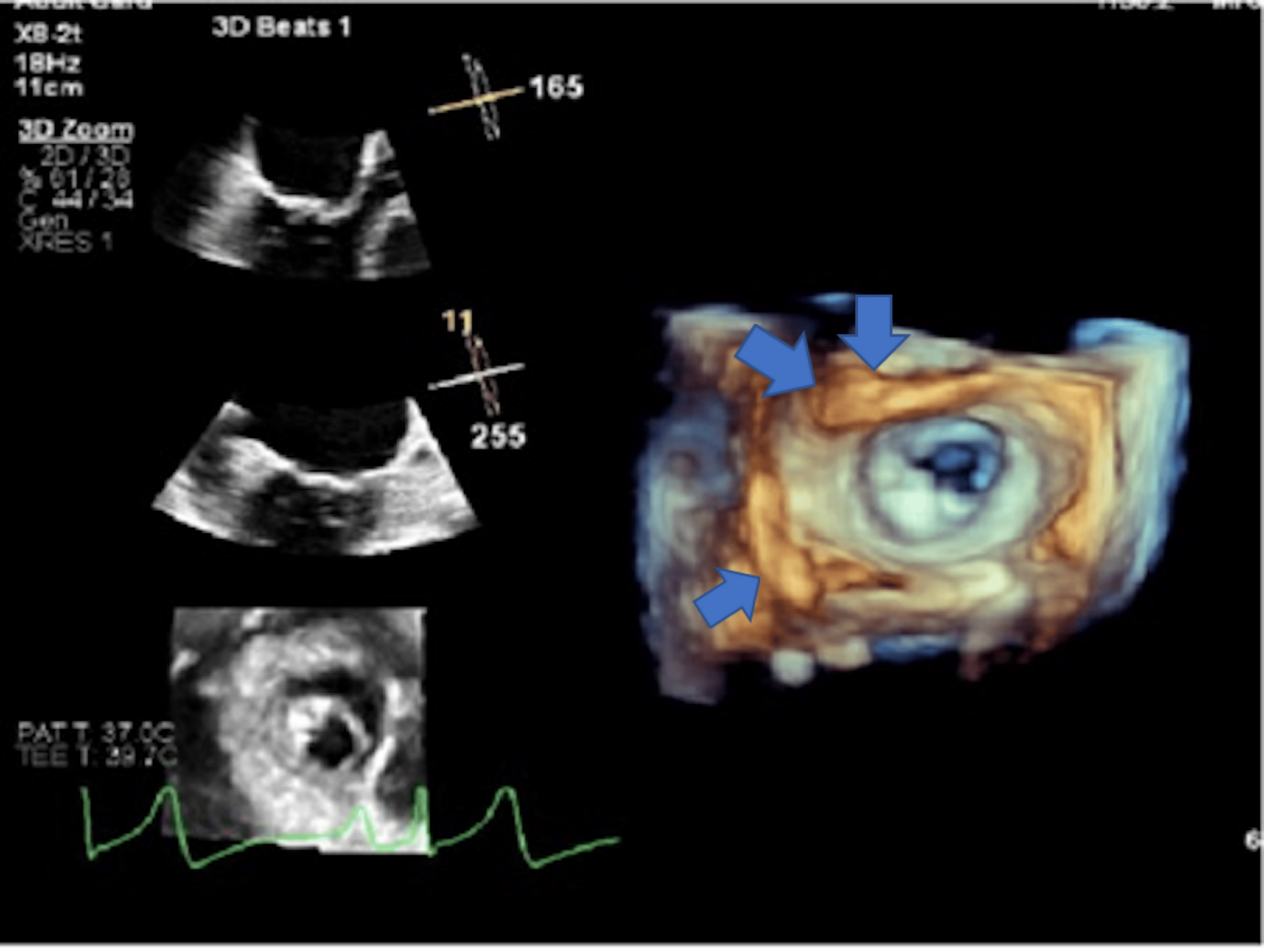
Transesophageal echocardiogram showing severe mitral stenosis with thickening of the mitral valve leaflets (blue arrows) (posterior > anterior leaflet) with a mitral valve area of 0.9 cm².
